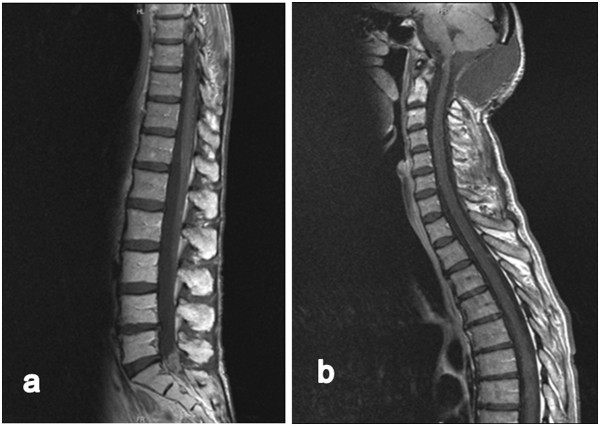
Sagittal T1-weighted magnetic resonance imaging images enhanced with gadopentetate dimeglumine, demonstrating stable disease without evidence of further tumor growth or new spinal or cranial manifestation 33 months after the first operation and 12 months after spinal irradiation (a, b).
